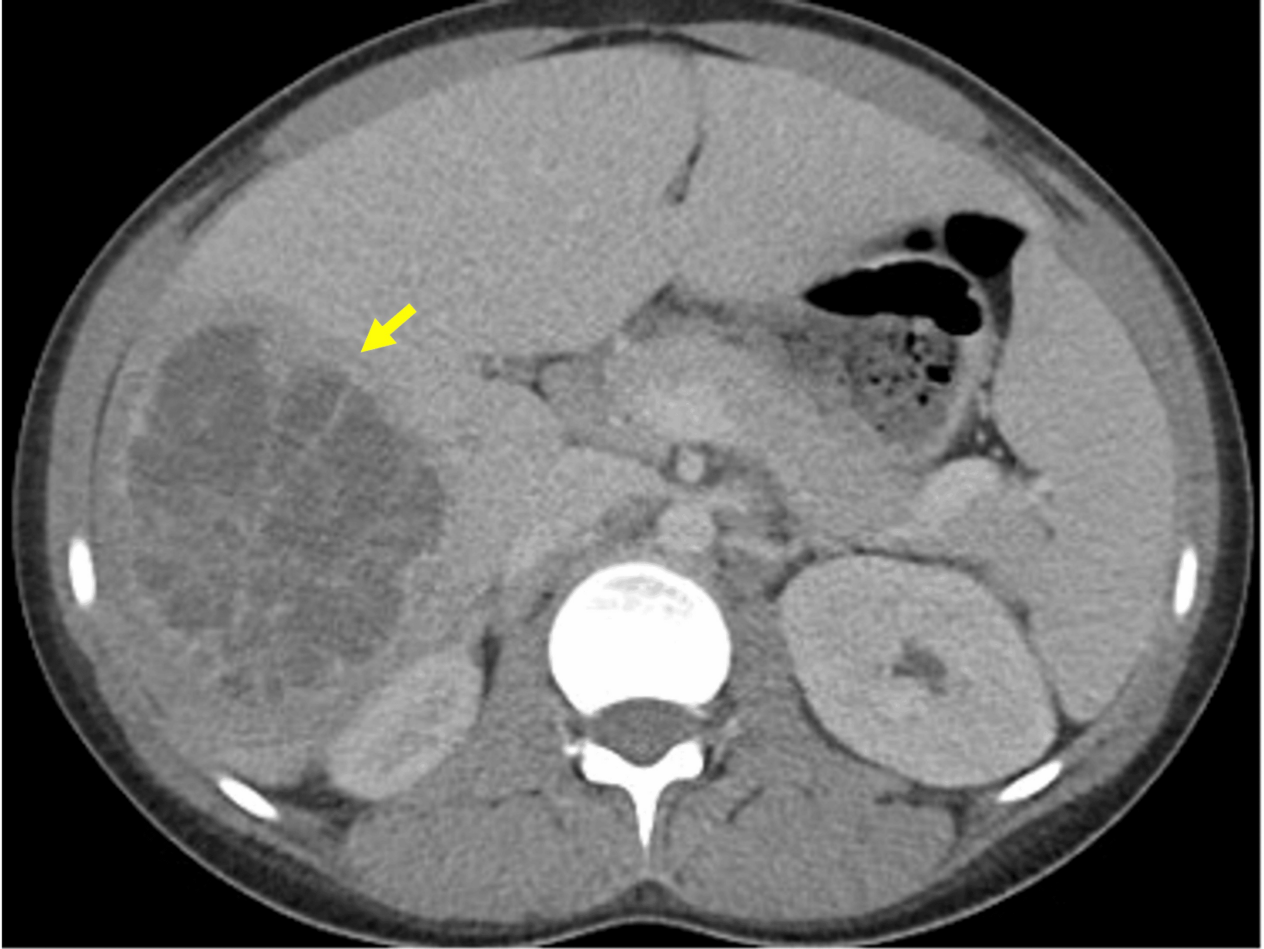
Abdominal CT with findings of right hepatic mass with surrounding edema
